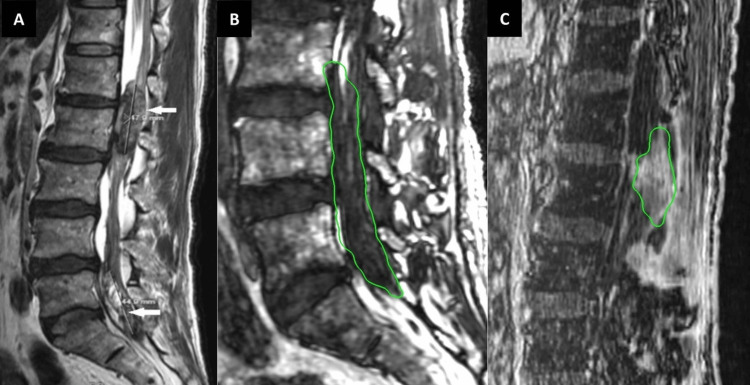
Fourteen-year follow-up: CyberKnife radiosurgery treatment for spinal metastases secondary to oligodendroglioma A: Preoperative sagittal T2-weighted lumbosacral spine MRI 14 years after the initial surgery presents two intradural enhancing masses (indicated by white arrows) in the lumbosacral spine. The rostral mass extends from L1 to L2, and the caudal mass extends from the L4-L5 disc space through the S1 spine. B: After 12 days of the last lumbosacral surgery, CK SRS was planned for the L4-S1 lesion. A marginal dose of 25.5 Gy was prescribed to the 83% isodose line. The maximum dose was 30.91 Gy (target contoured in green line). C: Two months after the last CK SRS treatment, another CK SRS was planned for recurrent L1-L3 lesions. This lesion was treated with a marginal dose of 25.5 Gy to the 80% isodose line. The maximum dose was 31.88 Gy. The gross tumor volume and clinical target volume were the same for these intradural lesions (target contoured in green line). CK SRS - CyberKnife stereotactic radiosurgery
